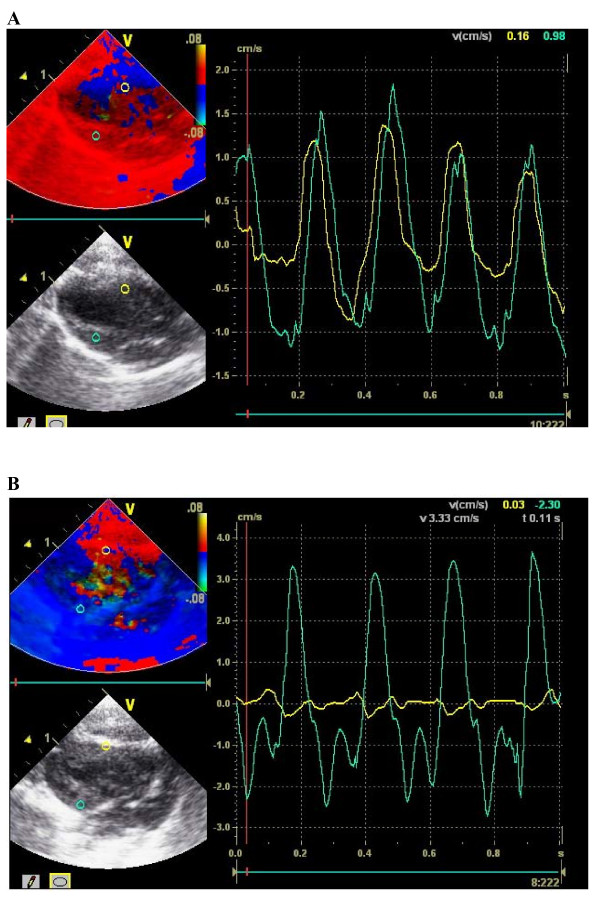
A and B: Graphic shows systolic movement of anteroseptal left ventricular wall (green curve) and posterior ventricular wall (yellow curve) by tissue doppler before (A) and one month after myocardial infarction (B). See "flat" postoperative anteroseptal signal.
